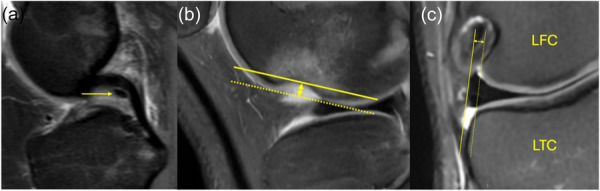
Investigated MRI signs. (a) pMFL presence; (b) deep LFNS; (c) LME. LFC, lateral femoral condyle; LFNS, lateral femoral notch sign; LME, lateral meniscus extrusion; LTC, lateral tibial condyle; MRI, magnetic resonance imaging; pMFL, posterior meniscofemoral ligament.
